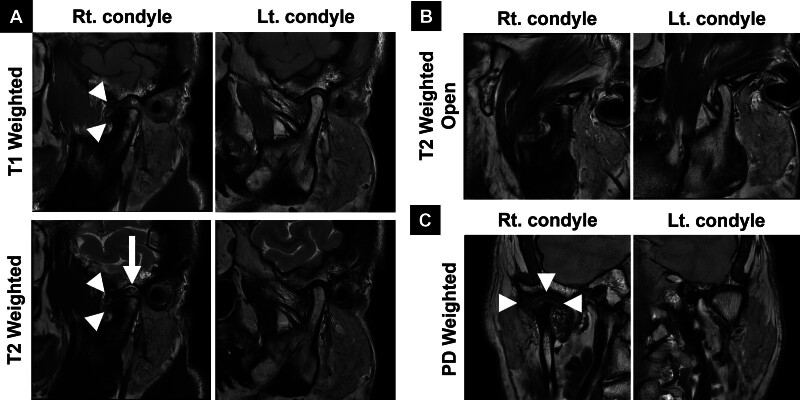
Preoperative magnetic resonance images (MRI). (A) T1 and T2-weighted oblique sagittal views in the closed-mouth position, showing the marrow and cortical continuity of osteochondroma with the underlying condyle head (arrowheads). Anteriorly displaced articular disc with joint effusion is visible in the T2 weighted images (arrows). (B) T2-weighted oblique sagittal view in the open-mouth position, showing reduction of the right articular disc, although its range of motion is slightly limited compared to the left TMJ. (C) Proton density (PD)-weighted coronal view in the closed-mouth position, showing the characteristic cartilage cap of the osteochondroma (arrowheads). TMJ = temporomandibular joint.
